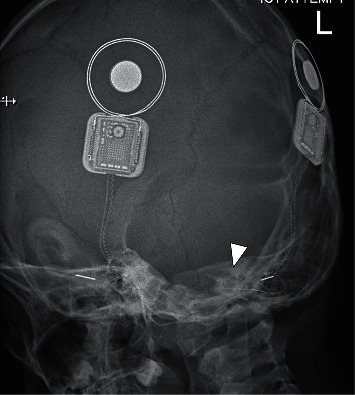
Lateral skull X-ray after trauma. The arrowhead points to the electrode which is curled in a 200-degree position. Comparing this with Figure 2 shows obvious migration of the electrode.
